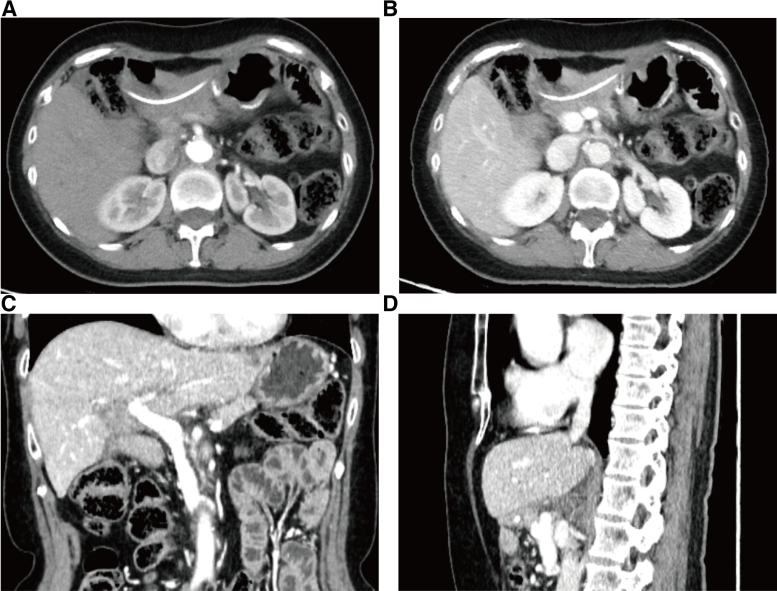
The CT scan image of the patient one year after the operation. (A,B) The CT scan images of the enhanced arterial (A) and venous phases (B) of the patient’s surgical area. (C,D) The CT scan images of the patient’s surgical area in the sagittal (C) and coronal positions (D). CT = computed tomography.
